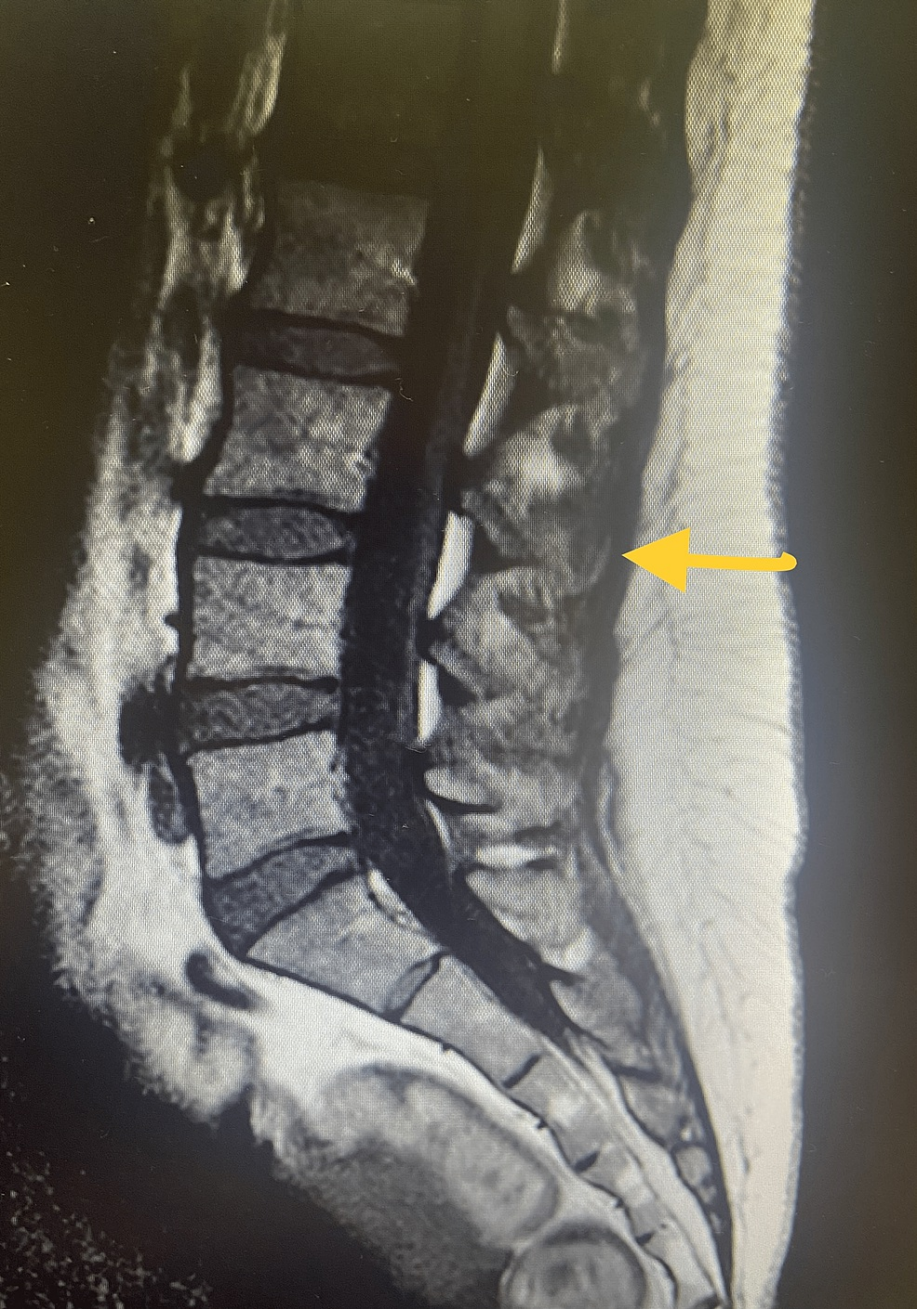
Lumbosacral spine MRI No abnormalities were detected on MRI. The yellow arrow points to the puncture site MRI: magnetic resonance imaging
